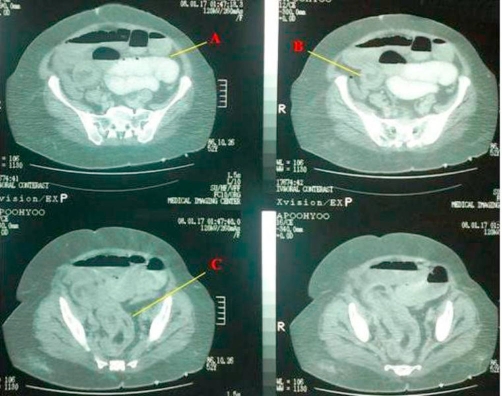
Computed tomography of abdomen showing (A) distention of small intestine and air-fluid level, (B) target lesion that characteristic for intussusceptions, (C) air between intussuscepted two lumens of ileum.
